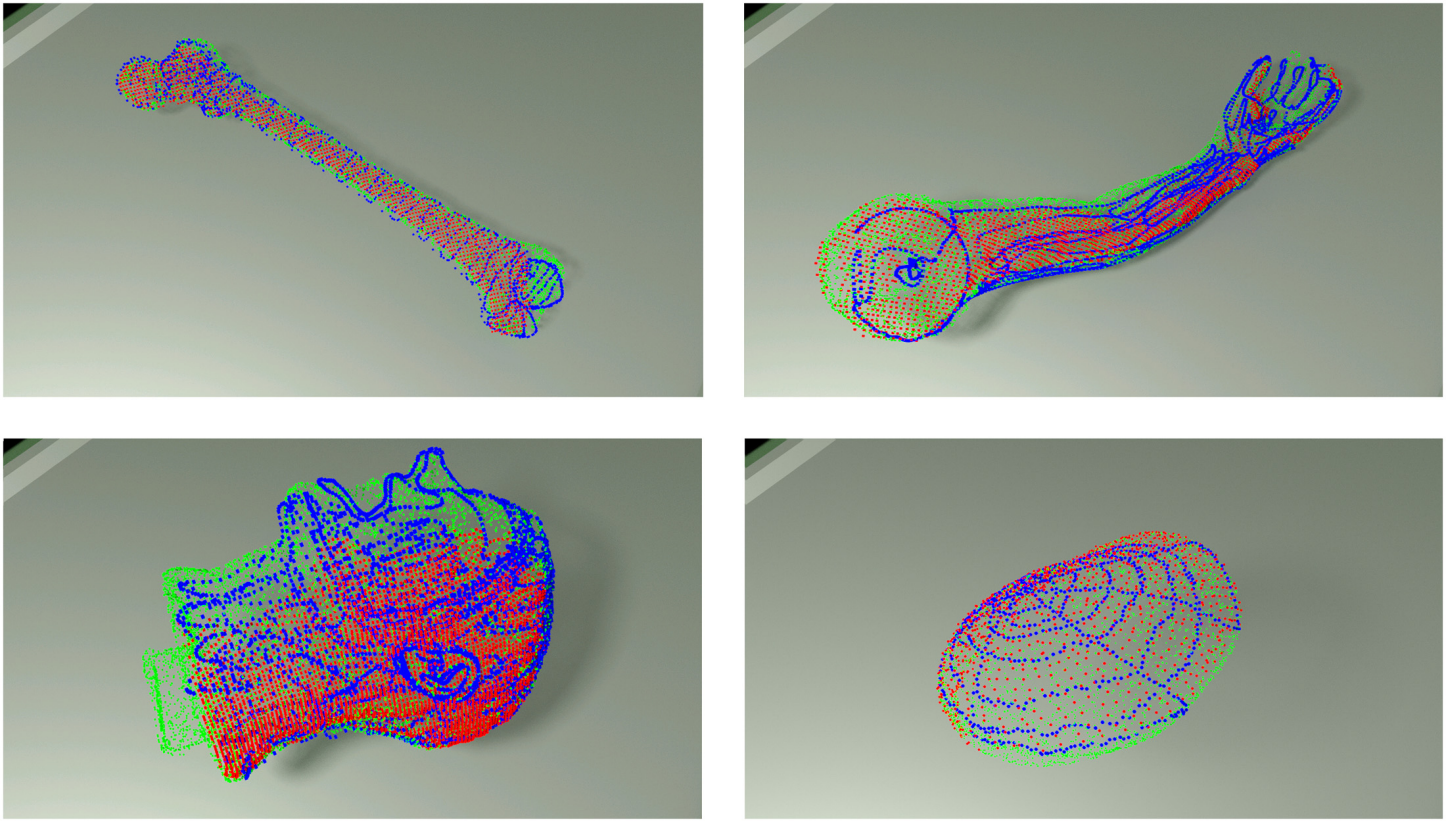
Visualisation of the registration results. ToF data (red), CT data (green), optical tracker data (blue).
